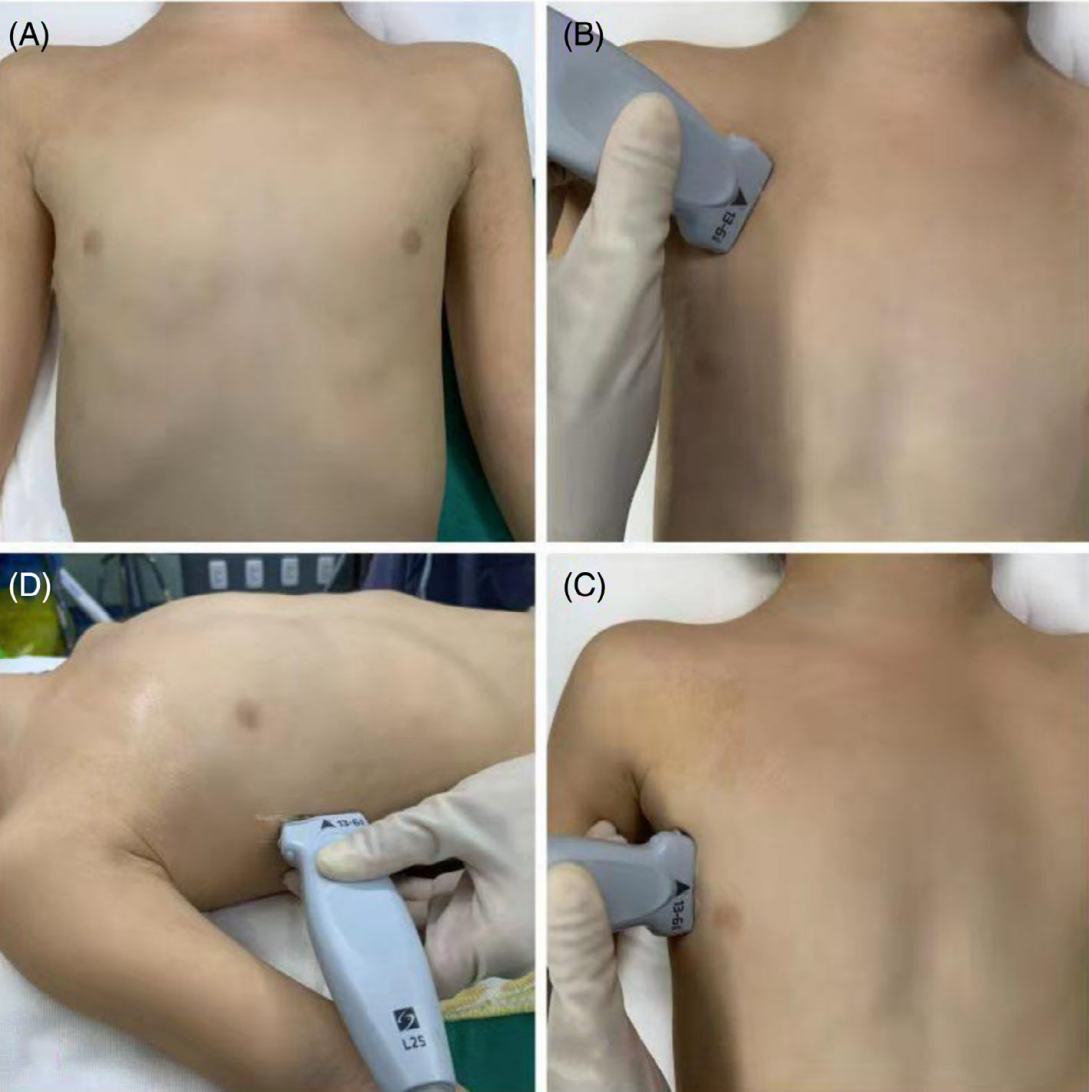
The placement of the probe at each ultrasound examination point. (A) The front of the child's chest; (B) The upper blue point; (C) The lower blue point; (D) The mid‐axillary line‐diaphragm point
